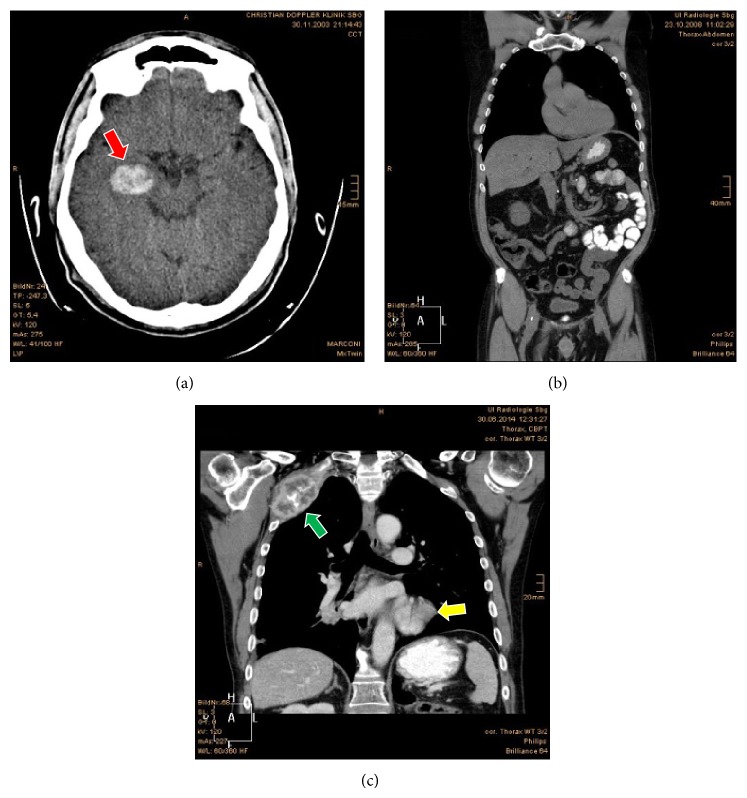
(a) Red arrow: brain CT axial view showing brain metastasis at the right temporal lobe in 2003. (b) Thorax, abdomen, and pelvis CT coronal view showing no evidence of metastasis after multiple metastasectomies. The scan was performed in 2008. (c) Recent (2014) chest CT coronal view showing a metastatic focus on the left lower lung lobe (yellow arrow) as well as an osteoclastic lesion involving the upper two ribs (green arrow).
